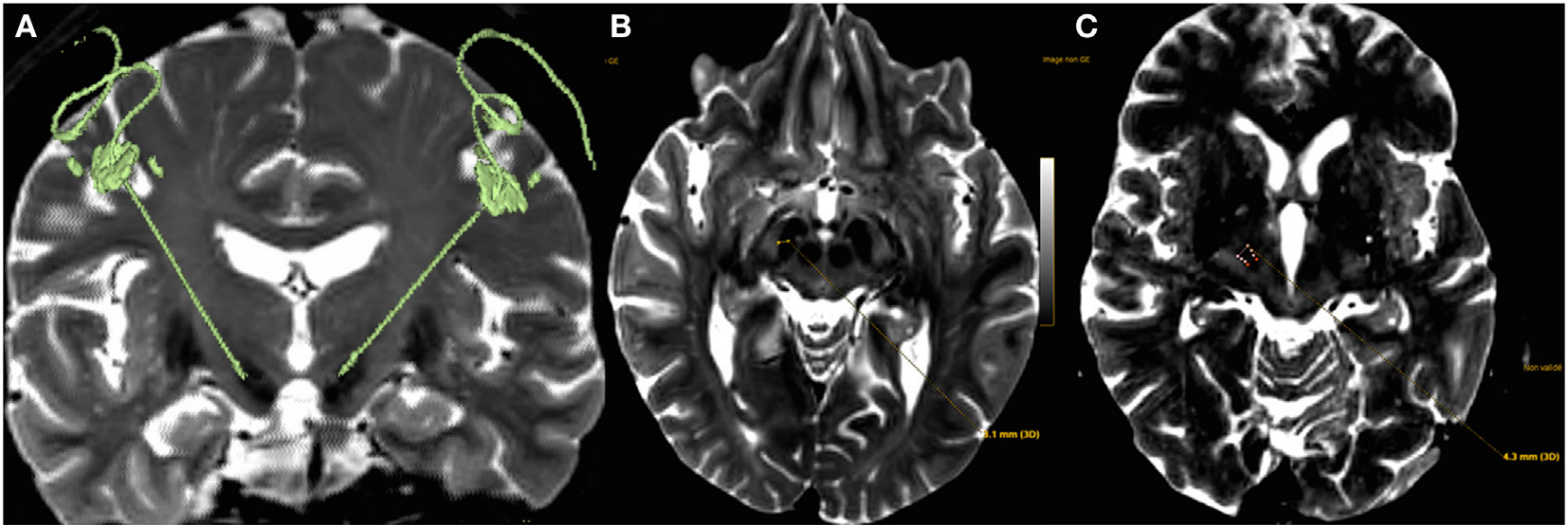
(A) 3D T2 sequence fused with postoperative CT showing electrodes placement within both subthalamic nucleus. (B) The first boundary of the “distance measurement tool” was adjusted on the center of the deep brain stimulation (DBS) contact visualized on the coronal plane of the CT image, here visible on an axial plane (T2-MRI; medial yellow point), extracted from the MRI-CT fusion; the lateral yellow point is the medial border of the corticospinal/corticobulbar tracts (CSBT) and was adjusted on the axial plane (MRI window). (C) For dorsal contacts (all four DBS contacts are represented medially, the most ventral one in red, the most dorsal one being the most anterior), the closest border of the CSBT to the electrode is no more medial but anterior or antero-medial.
